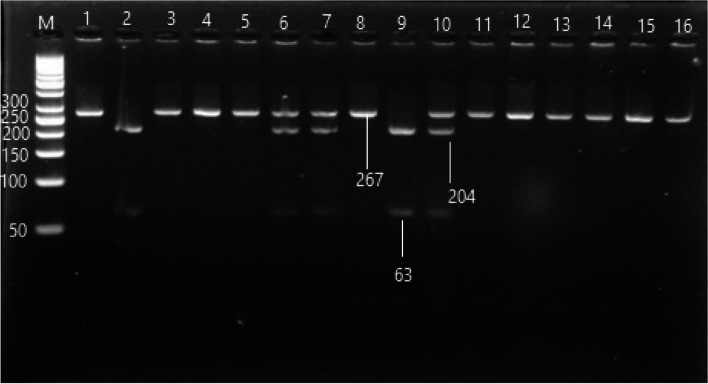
PCR–RFLP product of rs2228570 polymorphism, lane (M) 50 bp ladder, lanes (1,3,4,5,8,11,12,13,14,15,16) were CC genotype 267 bp, Lanes (6,7,10) were CT genotype 267, 204 and 63 bp, and lanes (2,9) were TT genotype 204 and 63 bp (Croped)
